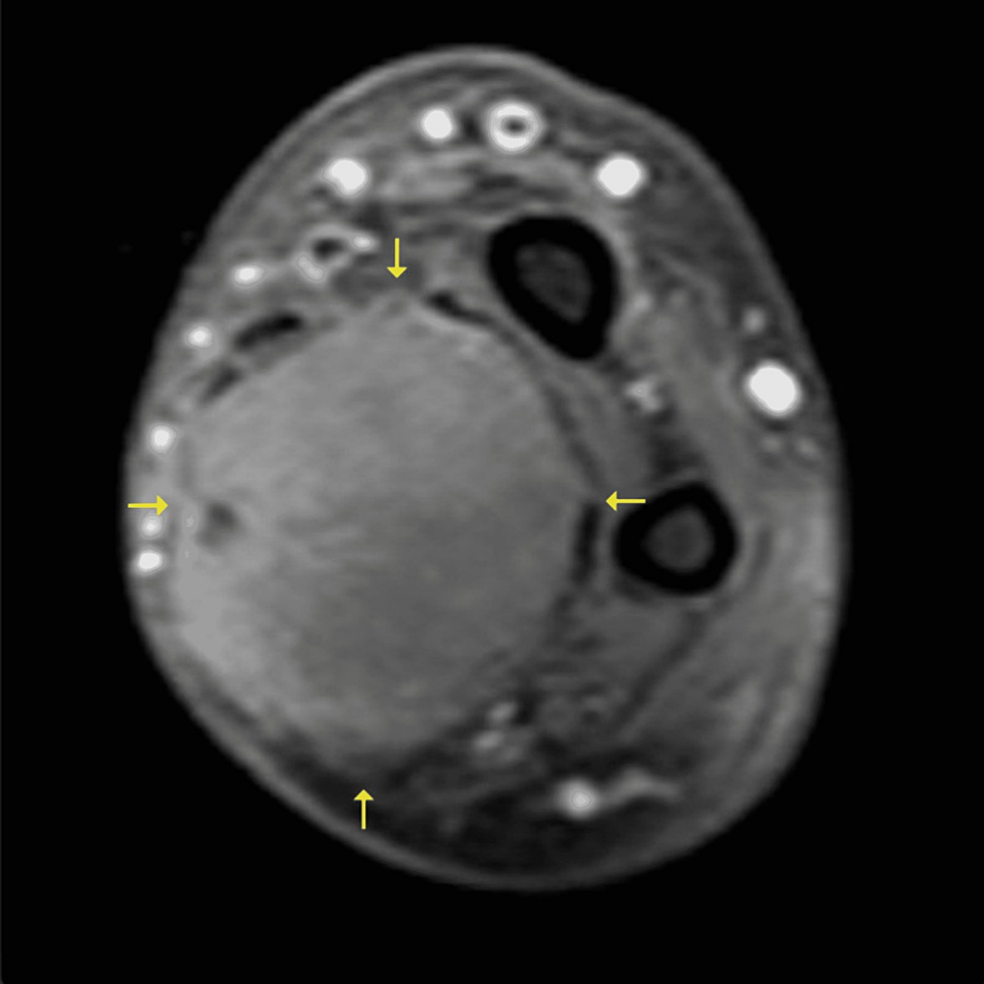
MRI showing a large, heterogeneously enhancing mass (arrows) in the right forearm with small, internal foci indicative of necrosis.
